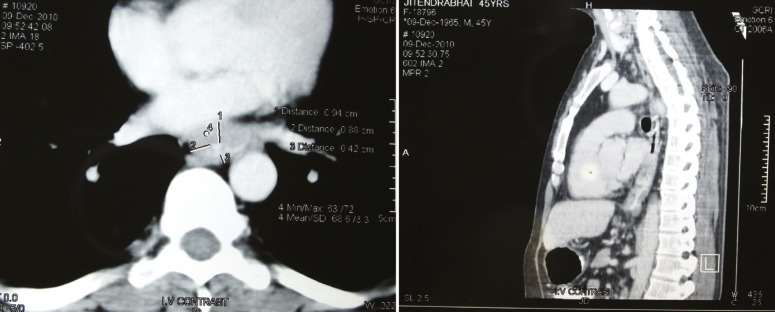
Computed tomography of the patient suggesting esophageal wall thickening without any lymphadenopathy
